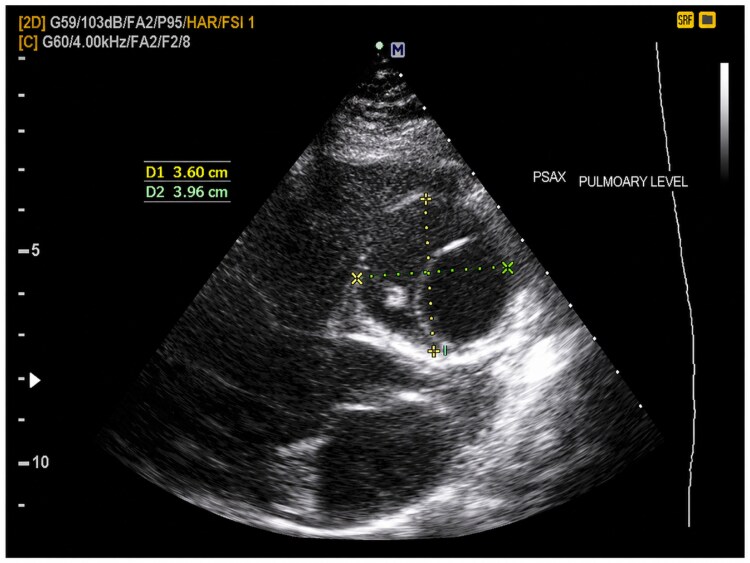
Transthoracic echocardiography, parasternal short-axis view at the pulmonary level, demonstrating a well-defined 3.6 × 3.9 cm intramyocardial cystic mass within the interventricular septum protruding towards the right ventricular cavity.
